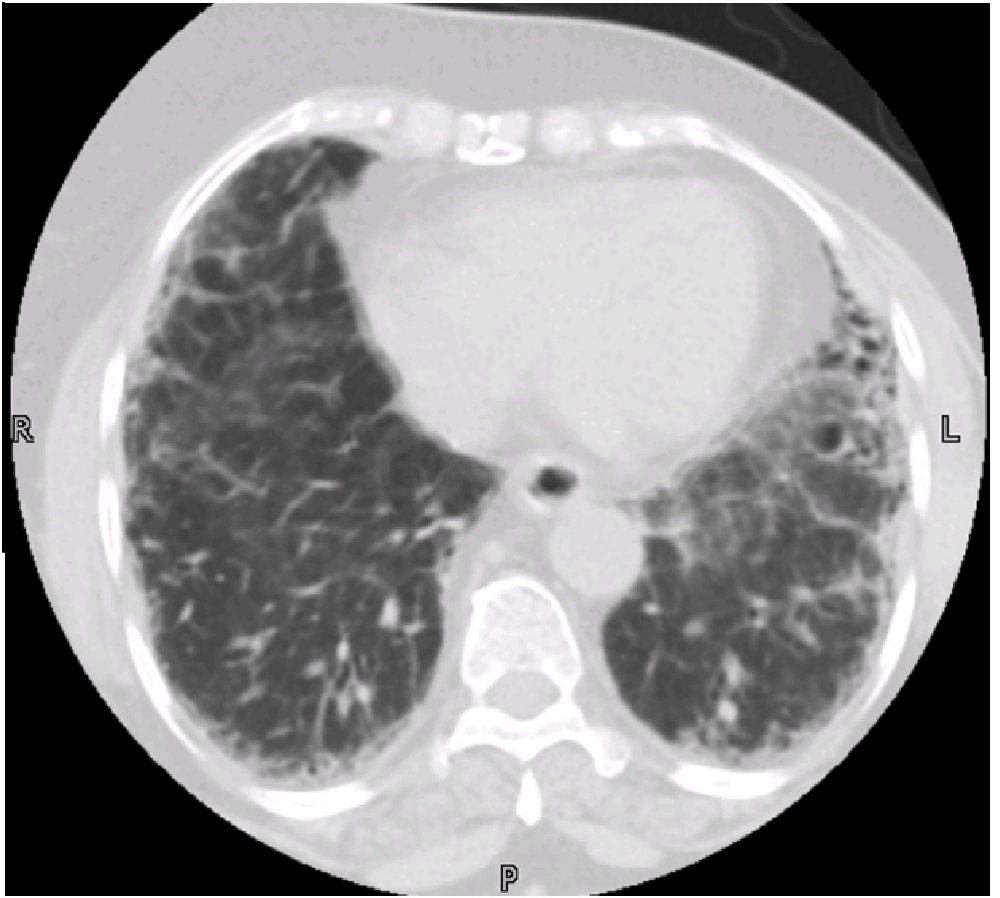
High-resolution computed tomography (HRCT) shows diffuse ground-glass opacities bilaterally
